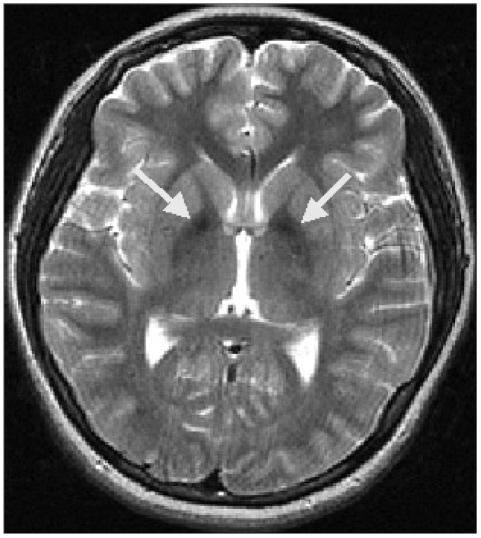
Magnetic resonance imaging. T2-weighted axial images showing areas of decreased signal intensity in the globus pallidus and the substantia nigra bilaterally.
